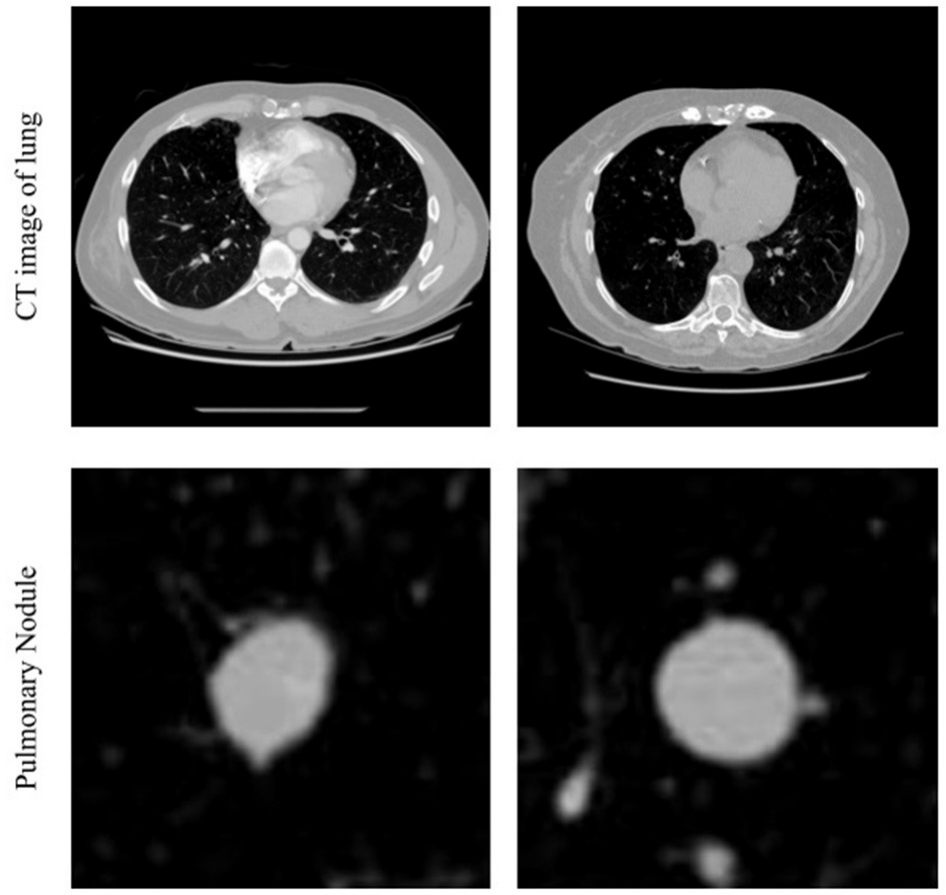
Example of CT images in lung and pulmonary nodule.
